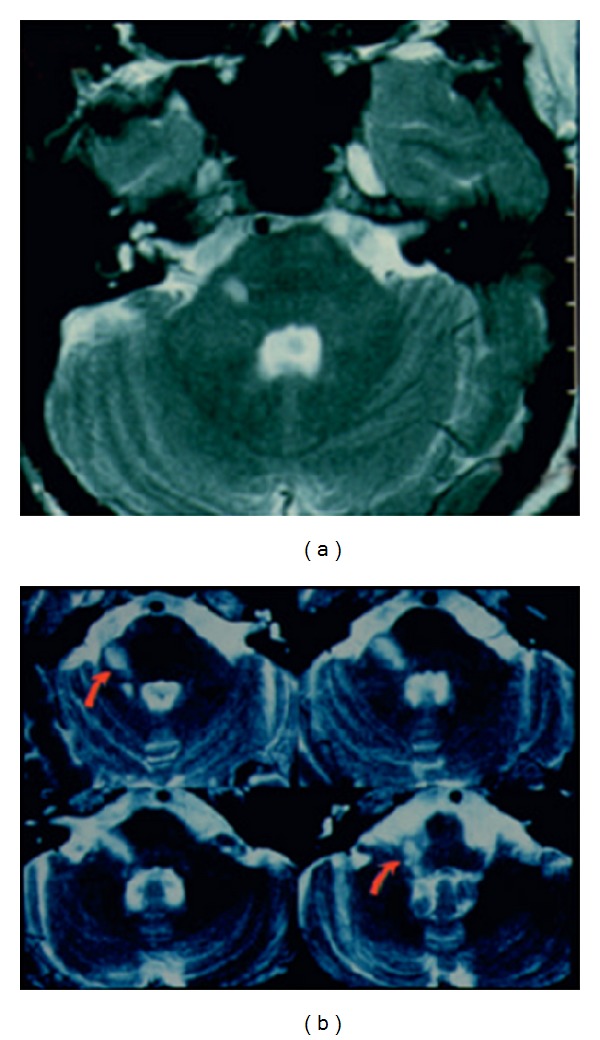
(a) (MRI-T2 image) Fascicular and nuclear lesion of the vestibular nerve due to a multiple sclerosis plaque mimicking vestibular neuritis. (b) (MRI-T2 image) Fascicular and nuclear lesion of the vestibular nerve due to a vascular lesion mimicking vestibular neuritis.
